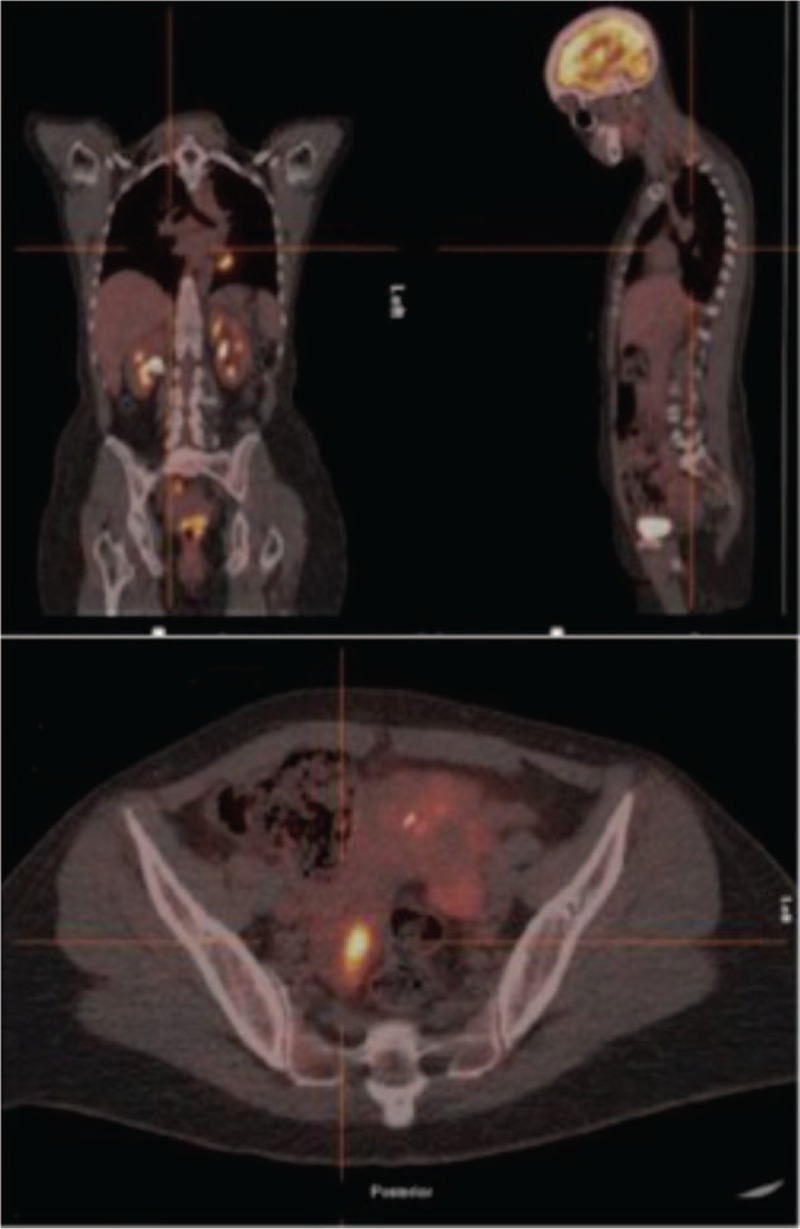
PET-CT with uptake in right hemipelvis.
